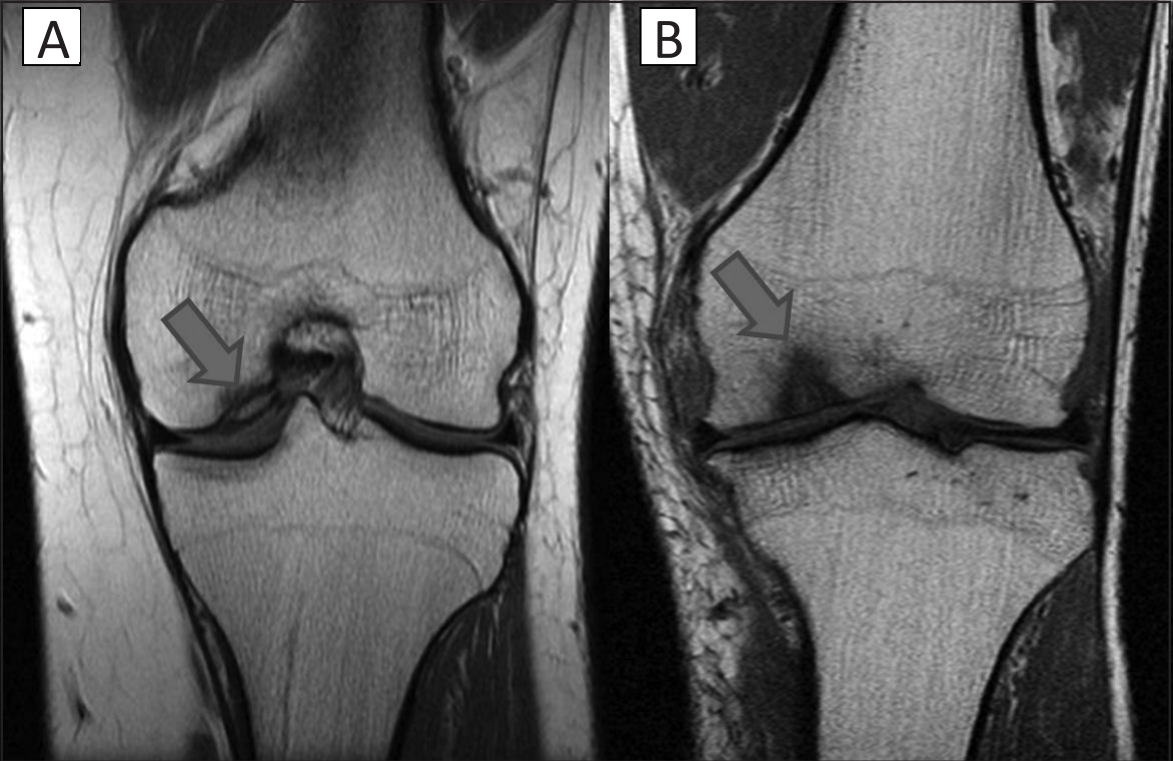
Coronal T1 weighted magnetic resonance imaging of the knee showing an area of osteochondritis dissecans affecting the medial femoral condyle (A) and an osteochondral lesion affecting the medial femoral condyle (B)
